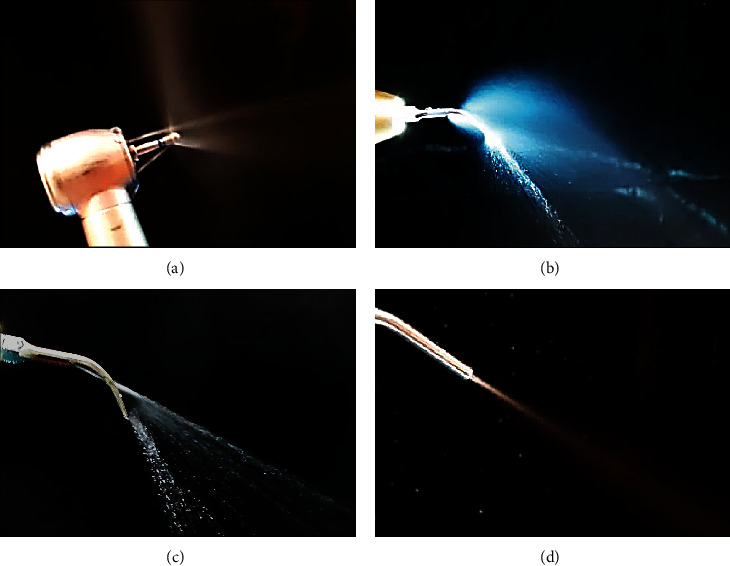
Aerosol-generating instruments used in the study. (a) Dental high-speed handpiece (KaVo, Standard head, Triple water spray, Berlin, Germany), with an irrigant flow rate of 50 mL/min. (b) Pneumatic scaler (AS2000, NSK, Shimohinata, Kanuma, Japan), with an irrigant flow rate of 55 mL/min. (c) Ultrasonic scaler (Cavitron Bobcat Pro, Dentsply, NY, USA), with an irrigant flow rate of 55 mL/min. (d) Air-water syringe, with an irrigant flow rate of 50 mL/min.
